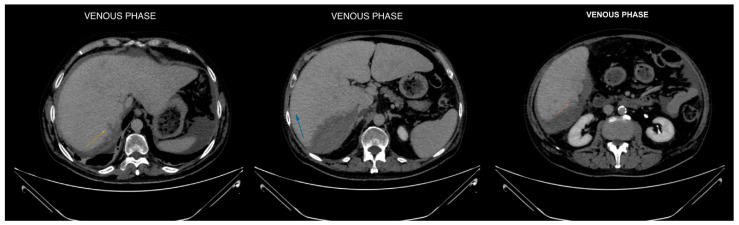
Axial CT images in venous phase focused on liver segments VI and VII, revealing hypodense subcapsular streaks (maximum extension of approximately 1.5 cm). These findings are consistent with hepatic lesions, likely indicative of a laceration [1,2].
